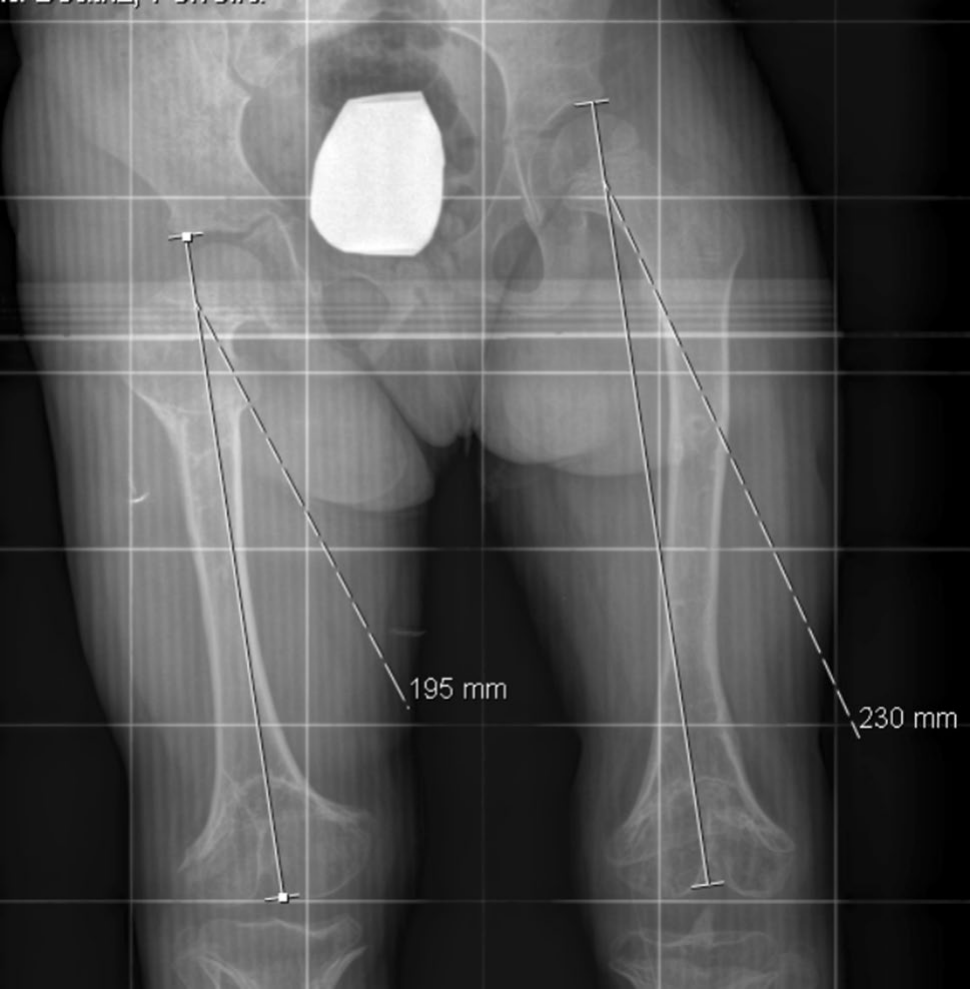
Preoperative X-ray with a right limb (femur) length discrepancy of 35 mm
